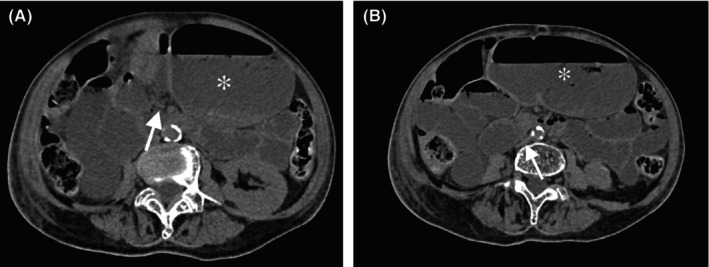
(A) Emergent plain abdominal computed tomography reveals paralytic ileus of the stomach (*) and small intestine, as well as narrowing of the superior mesenteric vein (arrow). (B) Emergent plain abdominal computed tomography reveals paralytic ileus of the stomach (*) and small intestine, as well as flattening of the inferior vena cava (arrow).
